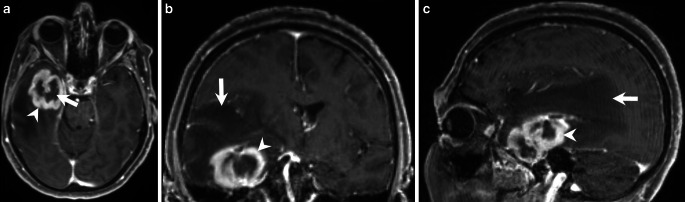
On axial (a), coronal (b), and sagittal (c) T1-weighted images taken after administration of gadolinium (Gd), the lesion showed distinct, giriform contrast enhancement (a–c, *arrowheads*) with signs of central necrosis (a, *arrow*). Please note the large perifocal edema (b, c, *arrow*)
